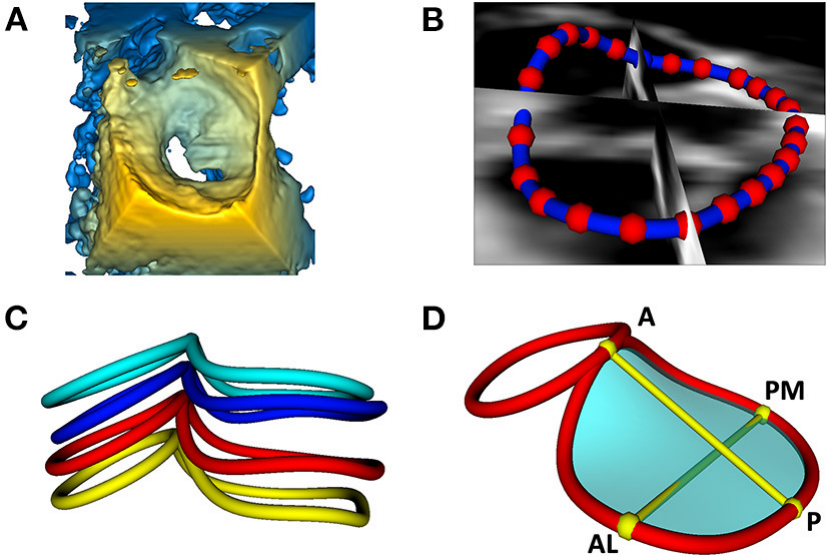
Visualization and modeling of mitral valve annuli. (A) Volume rendering of a 3DE of a mitral valve using the echo volume render module in SlicerHeart. (B) Curve creation of annular model of a mitral valve visualized through 2D slice intersections using valve annulus analysis module in SlicerHeart. (C) Visualization of mitral and aortic annular curves in all four phases of the cardiac cycle (top to bottom: End Diastole, Mid Diastole, Mid Systole, End Systole). (D) Quantification of mitral and aortic annuli using valve quantification module in SlicerHeart. 3DE, 3D echocardiogram; A, Anterior; P, Posterior; AL, Anterolateral; PM, posterior medial.
